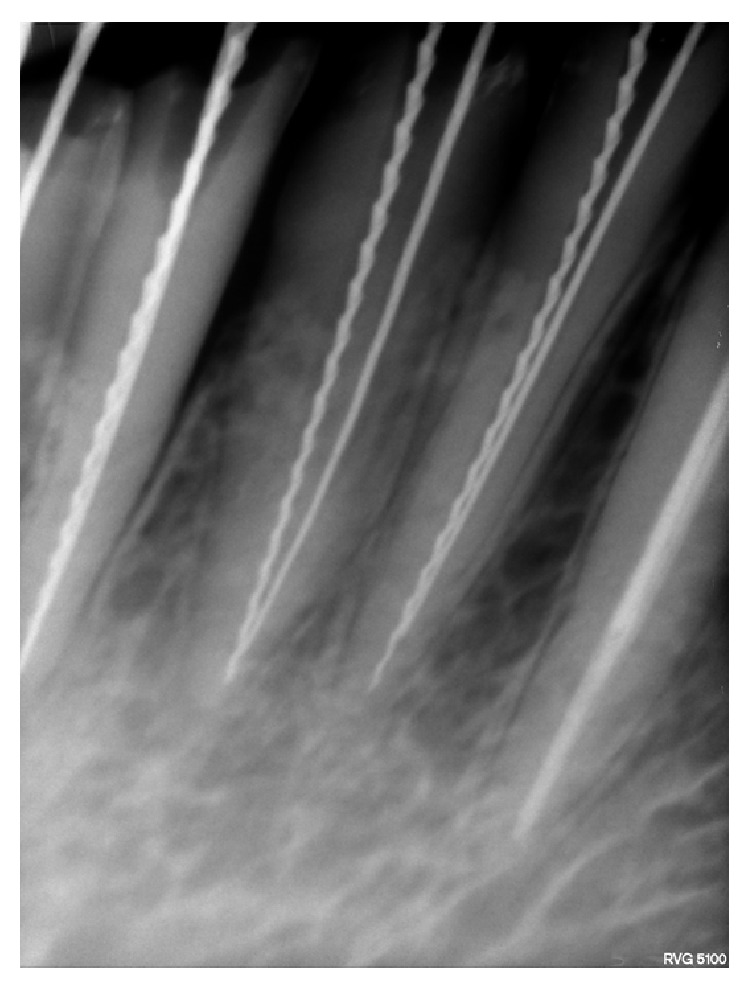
Radiograph showing two different instruments (“H” file and Reamer) in two canals in 41 and 42.
